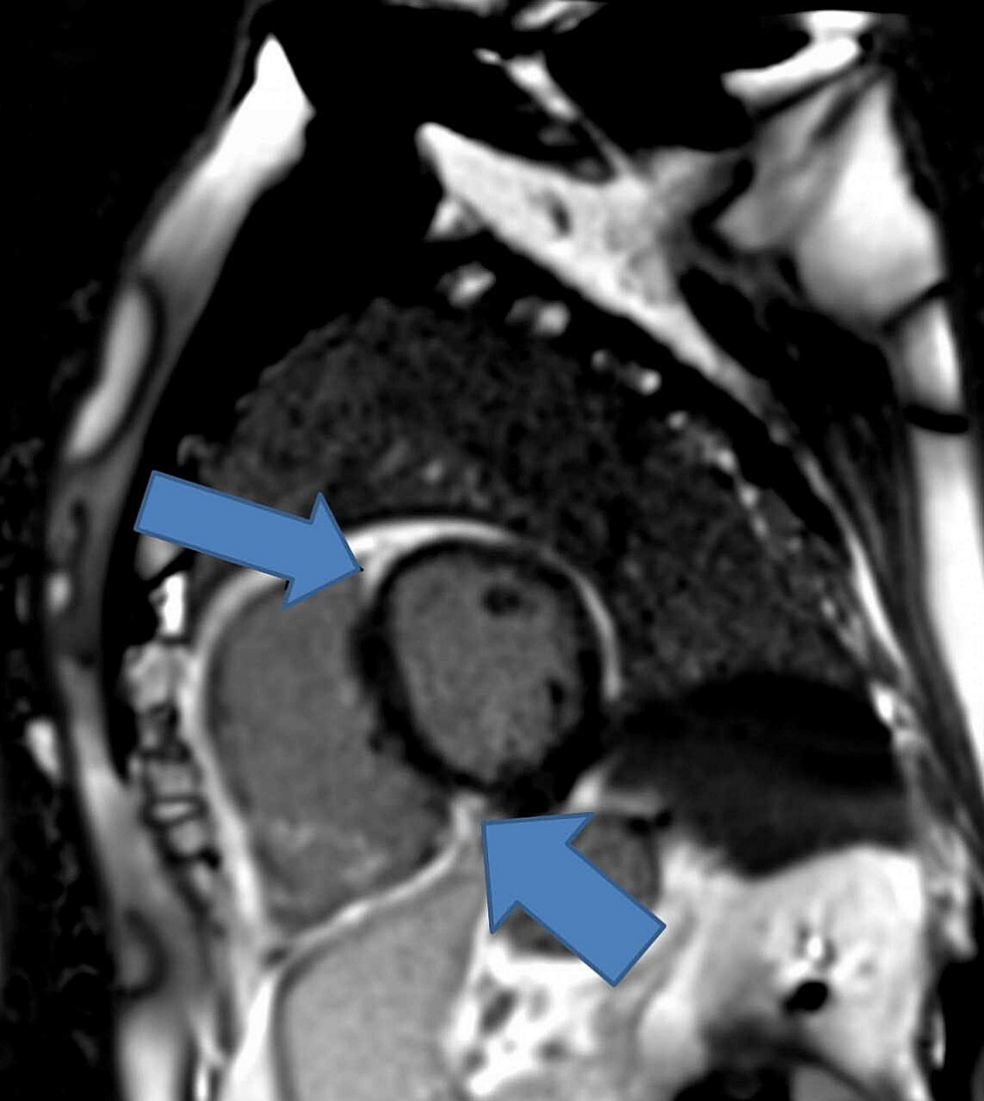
CMR showing LGE, a feature of ARVC (blue arrows) Arrhythmogenic right ventricular cardiomyopathy (ARVC); non-specific late gadolinium enhancement (LGE); cardiac magnetic resonance imaging (CMR)
